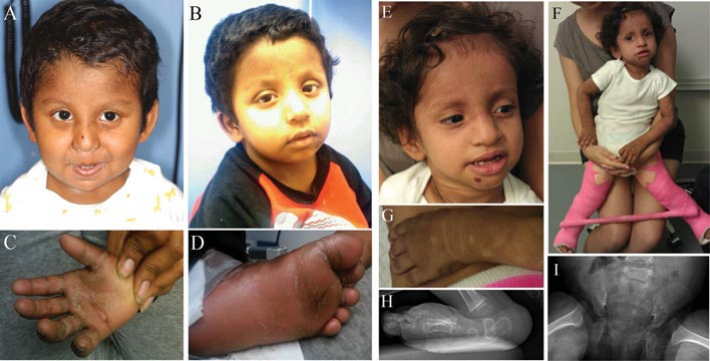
Patients with attenuated ARC syndrome. A–D: Patient AB. Aged (A) 3 years and (B) 5 years. Hyperkeratosis and lichenification of palm of right hand (C) and sole of left foot (D). E–I: Patient CD. Facies aged 3 (E); in plaster after corrective hip surgery (F). Hyperkeratosis, dorsum of right foot (G). Radiographs of right foot showing vertical talus (H) and of pelvis showing hip dislocation (I).
